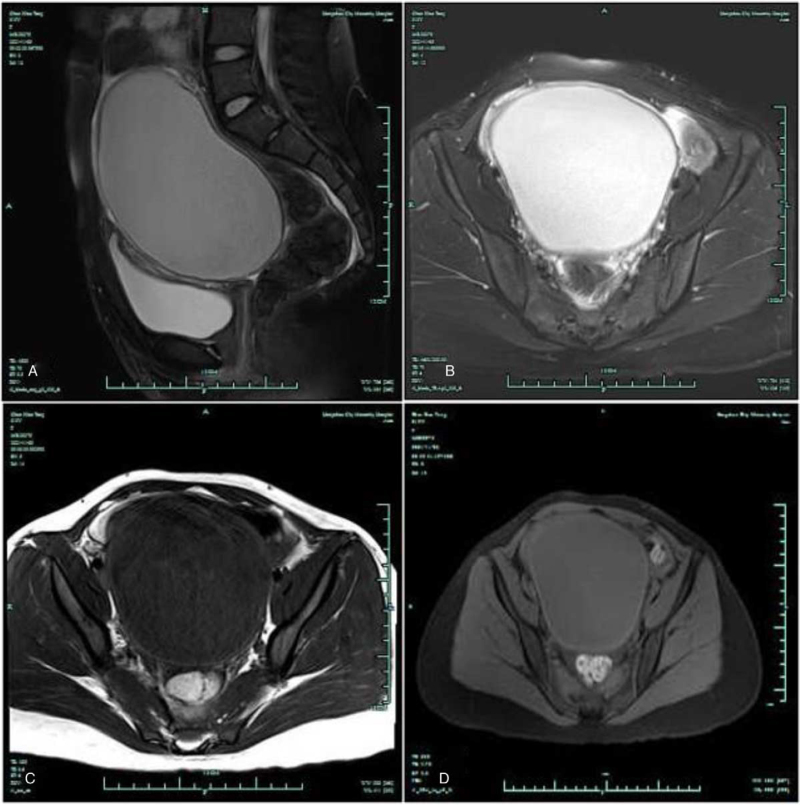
Magnetic resonance imaging in a sagittal image (A), a right ovarian mass with high signal intensity on T2-weighted images (B), low signal intensity on T1-weighted images (C), iso-hyper signal intensity on diffusion-weighted imaging (D). The white arrow shows the huge pelvic mass.
